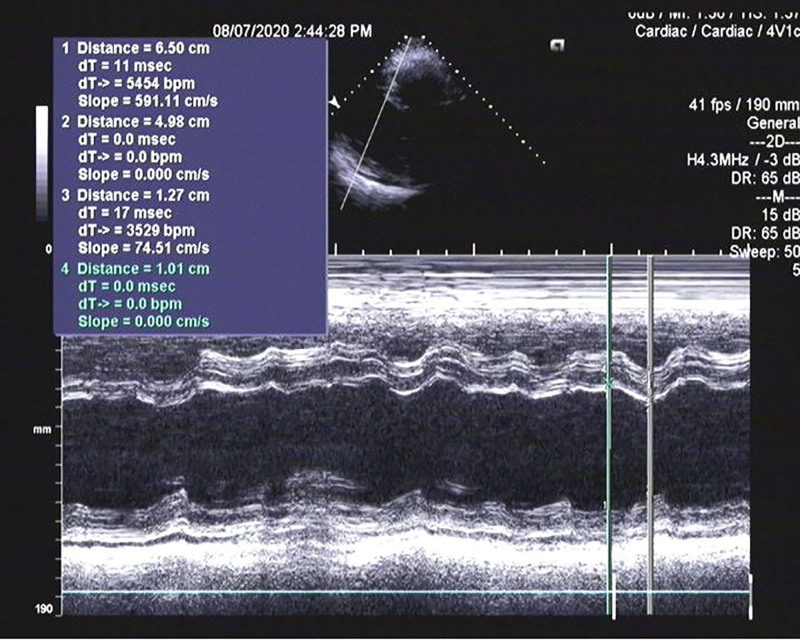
Echocardiogram showing a dilated and spherical left ventricle: Left ventricular end-diastolic diameter: 65 mm and left ventricular end-systolic diameter: 49.8 mm.
